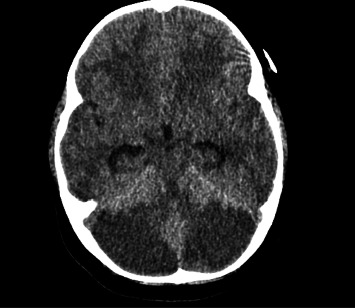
Initial head CT (12–24 h after presumed ingestion) showing diffuse edema throughout the cerebellum with no acute hemorrhage.
